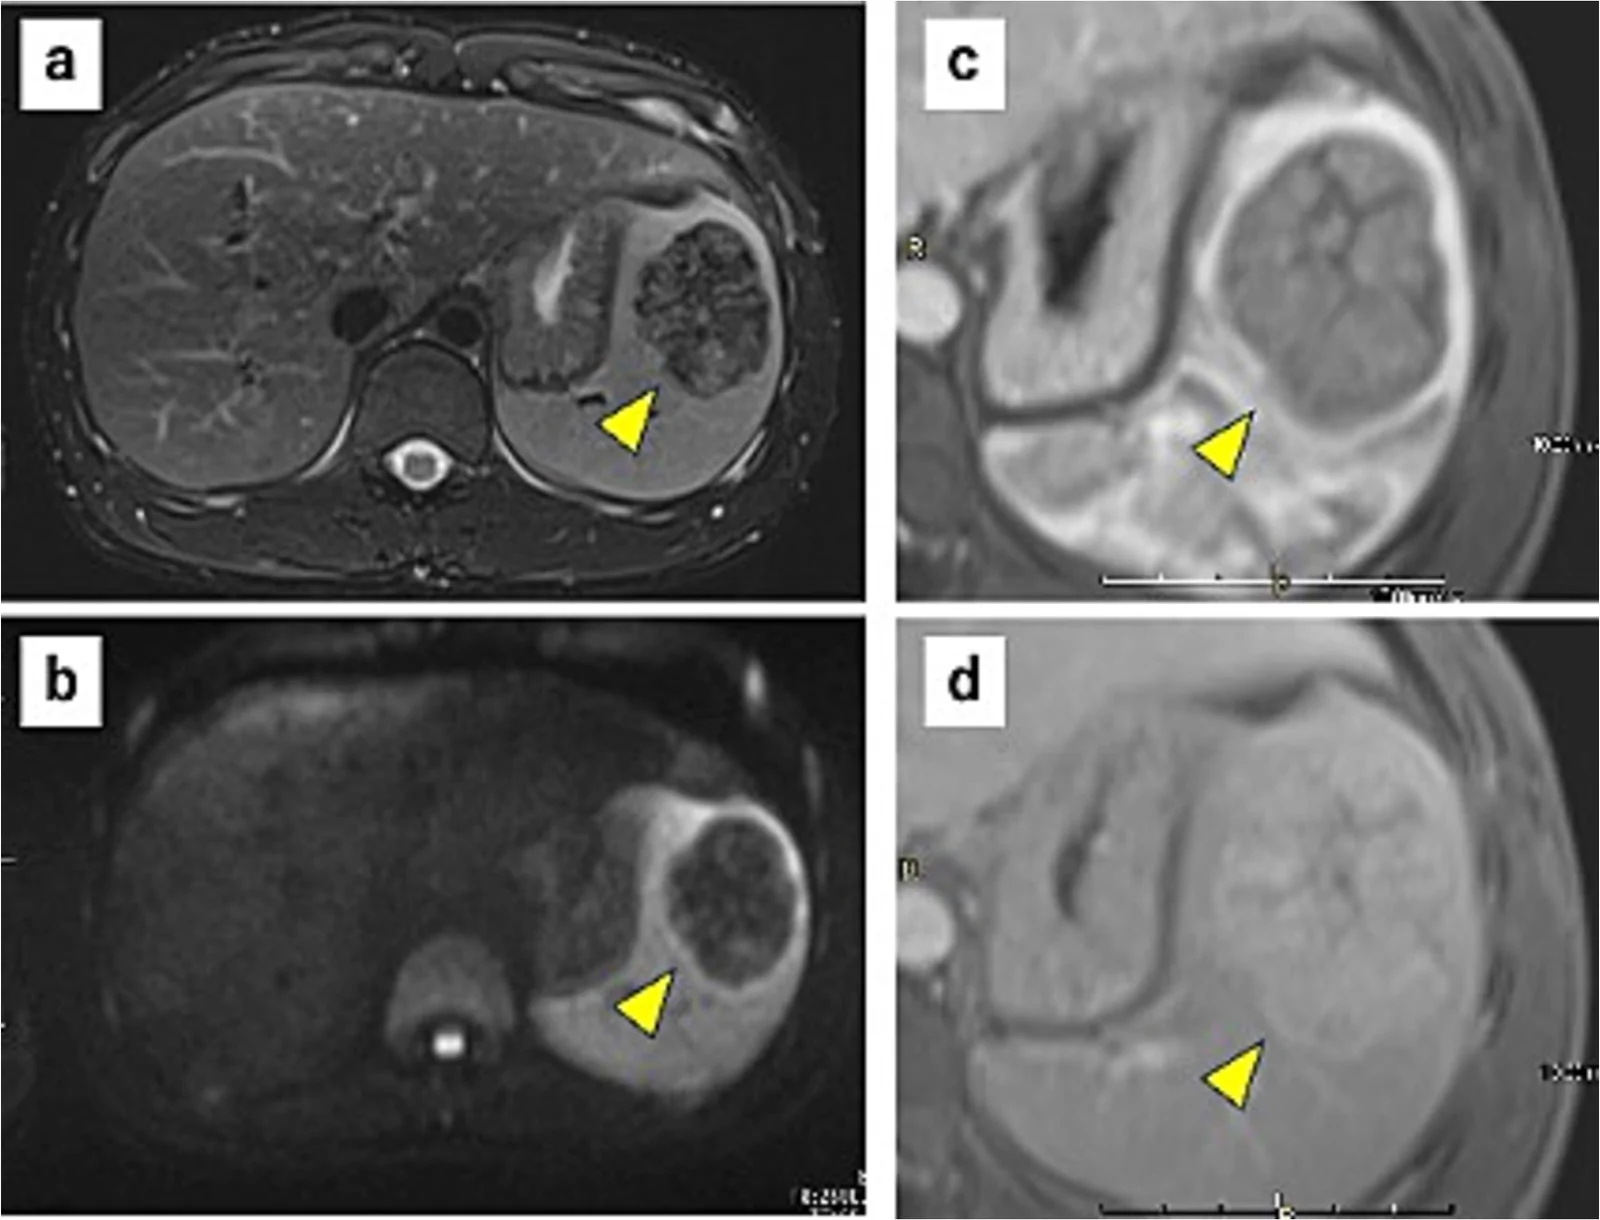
MRI. (a) T2-weighted image showing heterogeneous low-to-isointense signal intensity. (b) Diffusion-weighted image demonstrating low signal intensity. (c) Arterial phase and (d) equilibrium phase contrast-enhanced MRI images show a spoke-wheel–like enhancement pattern similar to that observed on CT.
